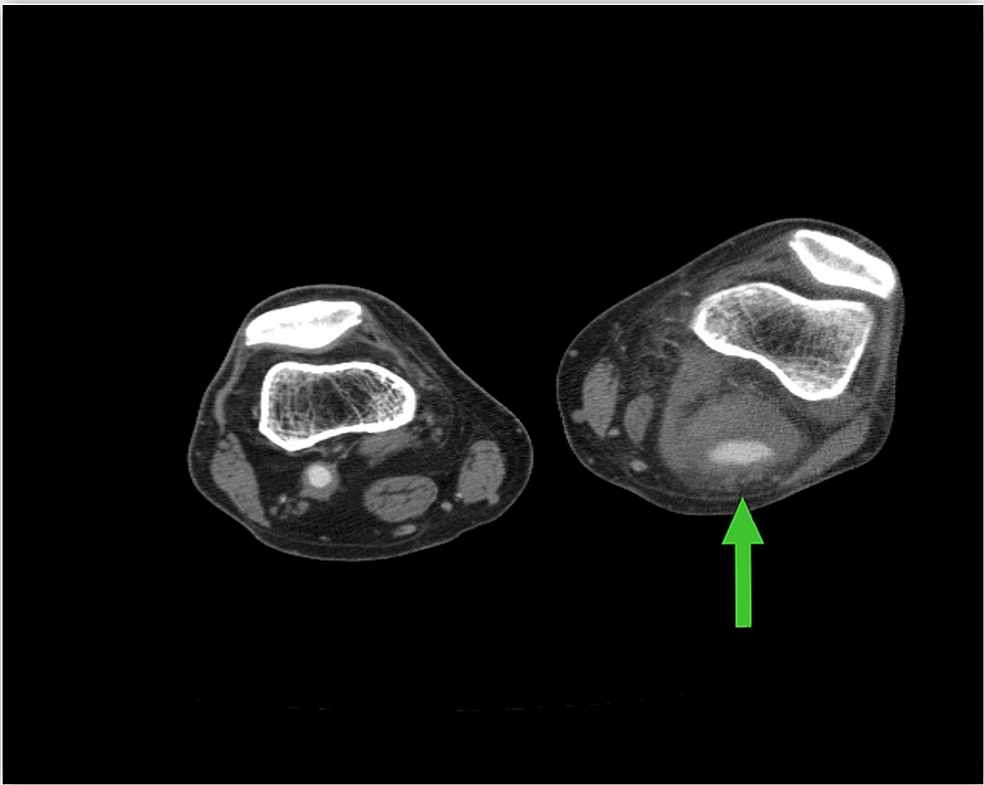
Preoperative CT scan of lower extremities The image demonstrates a left popliteal fossa mass consistent with a popliteal artery aneurysm with a patent lumen and large laminated thrombus (arrow) CT: computed tomography
